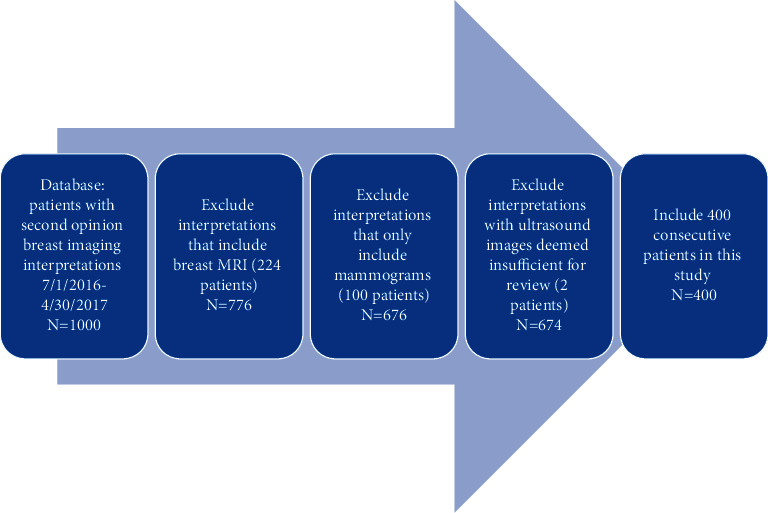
Inclusion and exclusion criteria leading to the inclusion of 400 consecutive patients with second opinion breast ultrasound review.
